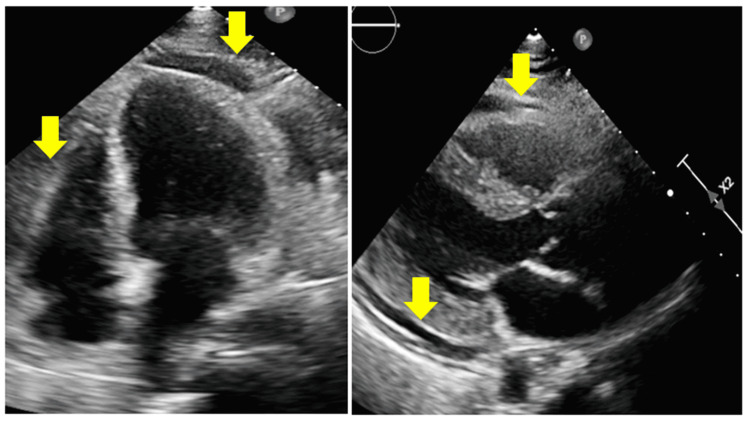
Initial echocardiographic findings showing RV diastolic collapse and moderate-to-large pericardial effusion suggestive of cardiac tamponade (yellow arrows).
